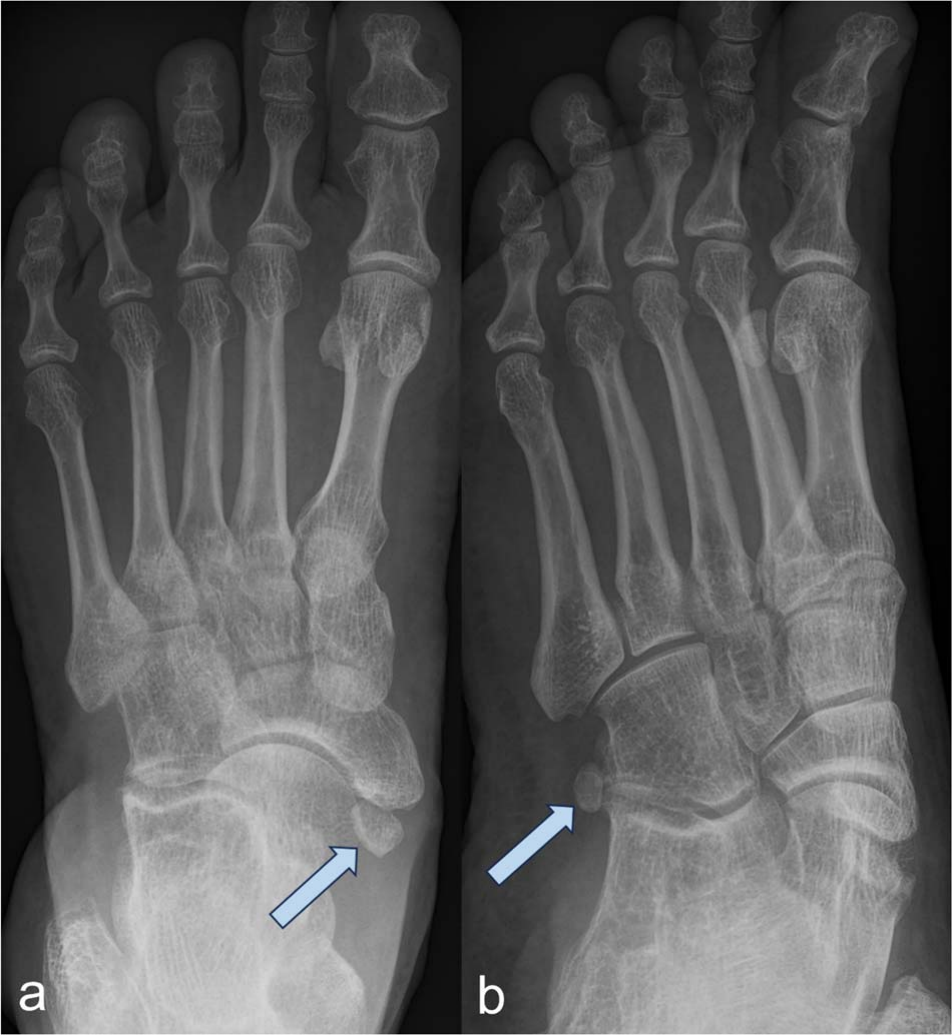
Image of an Os tibiale externum Type II in the anterior–posterior (a) and oblique (b) radiographic view; the ossicle is marked with an arrow.
